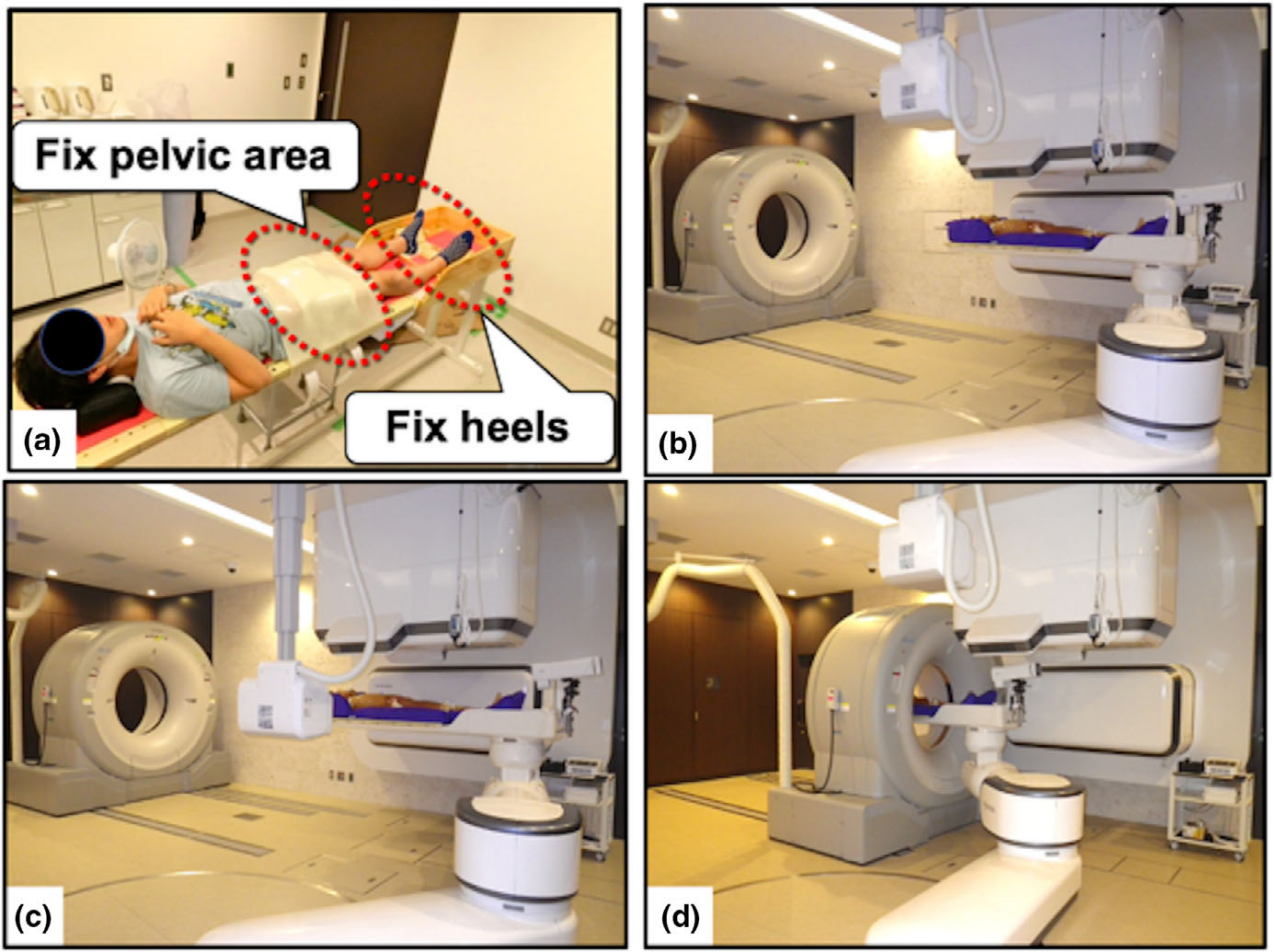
(a) Immobilization of a patient with prostate cancer. The patient moved to the treatment position (b), and we acquired a two‐dimensional (2D) orthogonal x‐ray image and compared bone structure between the X‐ray and planning computed tomography (CT) images. The patient was moved to the correct position automatically (c). Then, we acquired in‐room CT images and verified that patient set‐up was acceptable (d).
